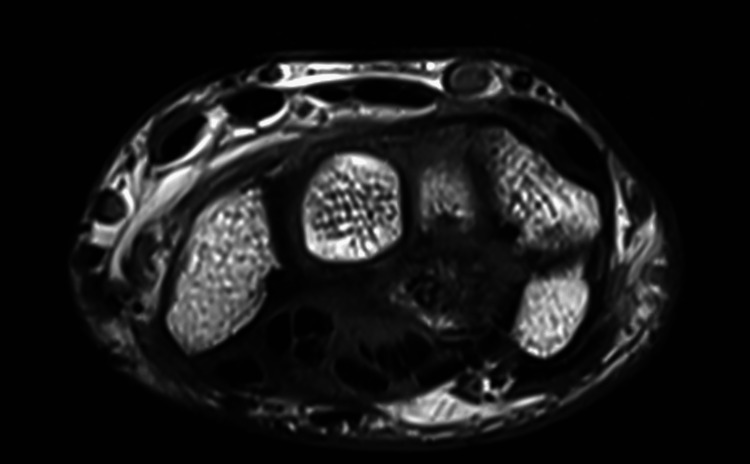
MRI showing the loss of normal smooth contour with cortical irregularity and marrow edema at the superior articular portion
